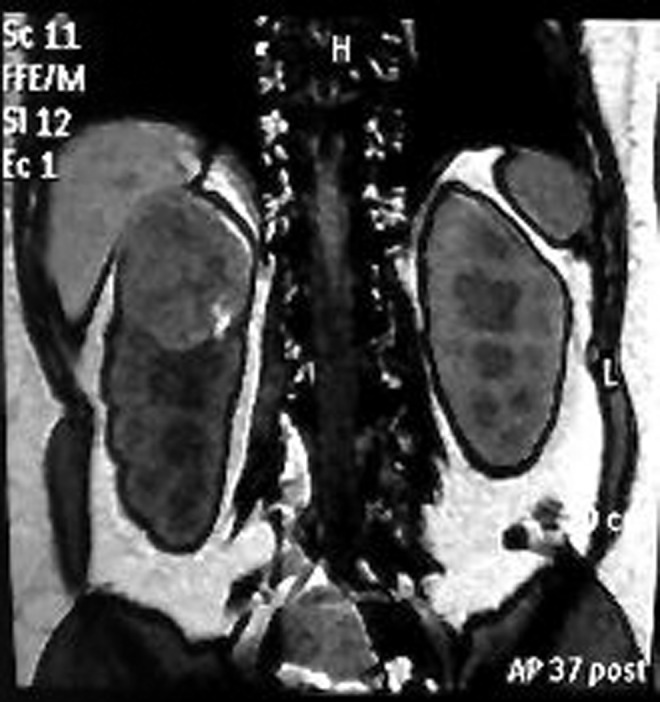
Preoperative MRI of the patient.
